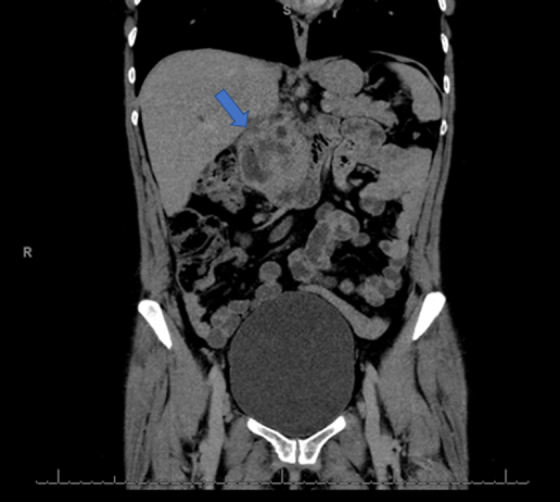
Coronal CT image revealing a 4.6 cm by 3.6 cm hypoattenuating mass (arrow) in the HOP.
